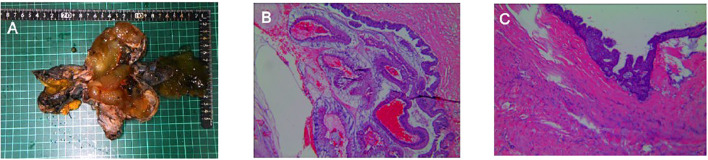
Six-month postoperative follow-up MRI of the patient. (A, B) MRI scans showing multiple lesions in the abdominal cavity and peritoneum. (C) The right testicular mass has been excised, demonstrating postoperative changes without any evidence of residual tumor or recurrence.
